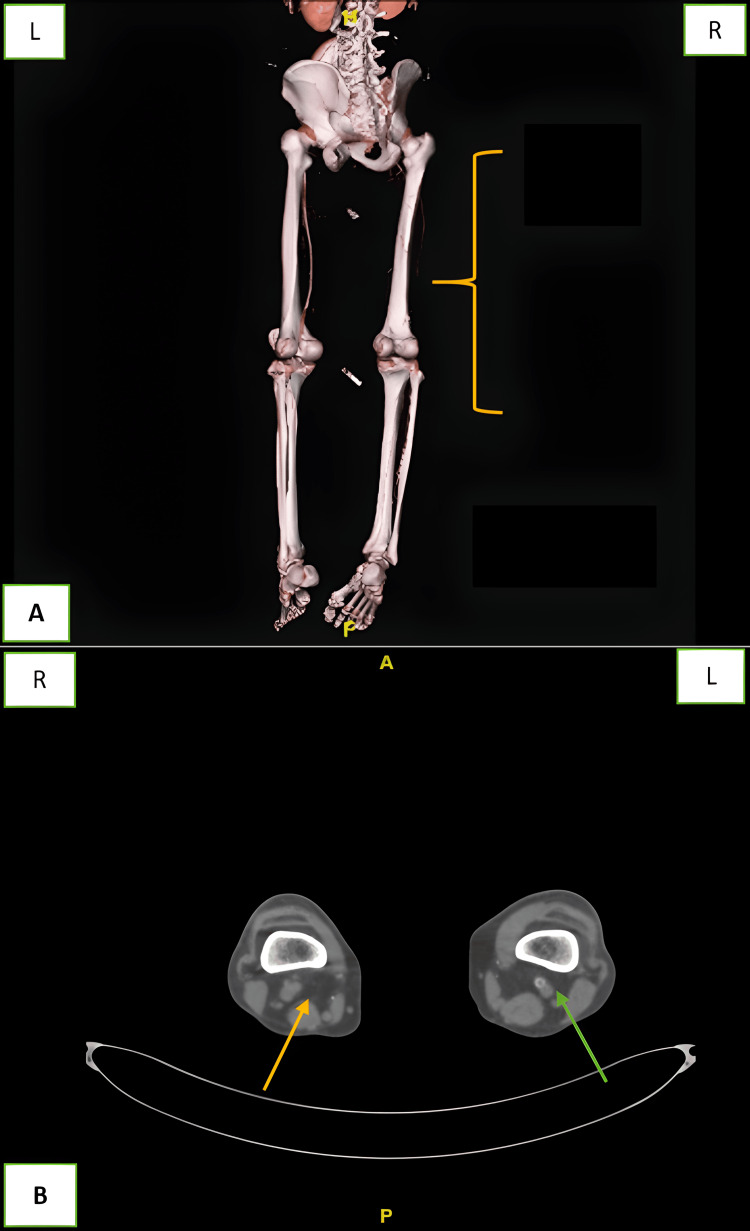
CT angiography of lower limbs. (A) 3D view showing that the right superficial femoral artery (SFA) is not opacified till the popliteal artery (yellow mark) due to thrombosis. (B) Axial view of the proximal thigh showing that the right SFA is not opacified (arrow yellow) but the left SFA is opacified (arrow green). R: right, L: left, A: anterior, P: posterior.
